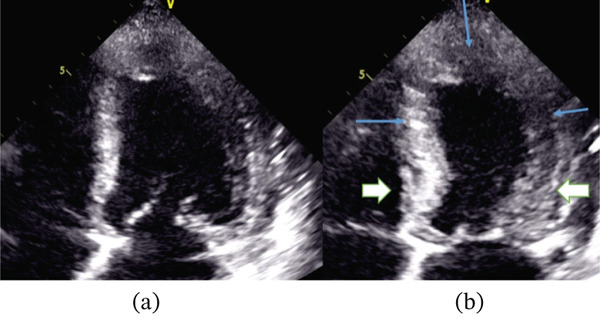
Transthoracic echocardiography showing apical four‐chamber view of the left ventricle in diastole (a) and systole (b) showing good basal contractions (black arrows) but poor mid and apical segment contractions (blue arrows).
